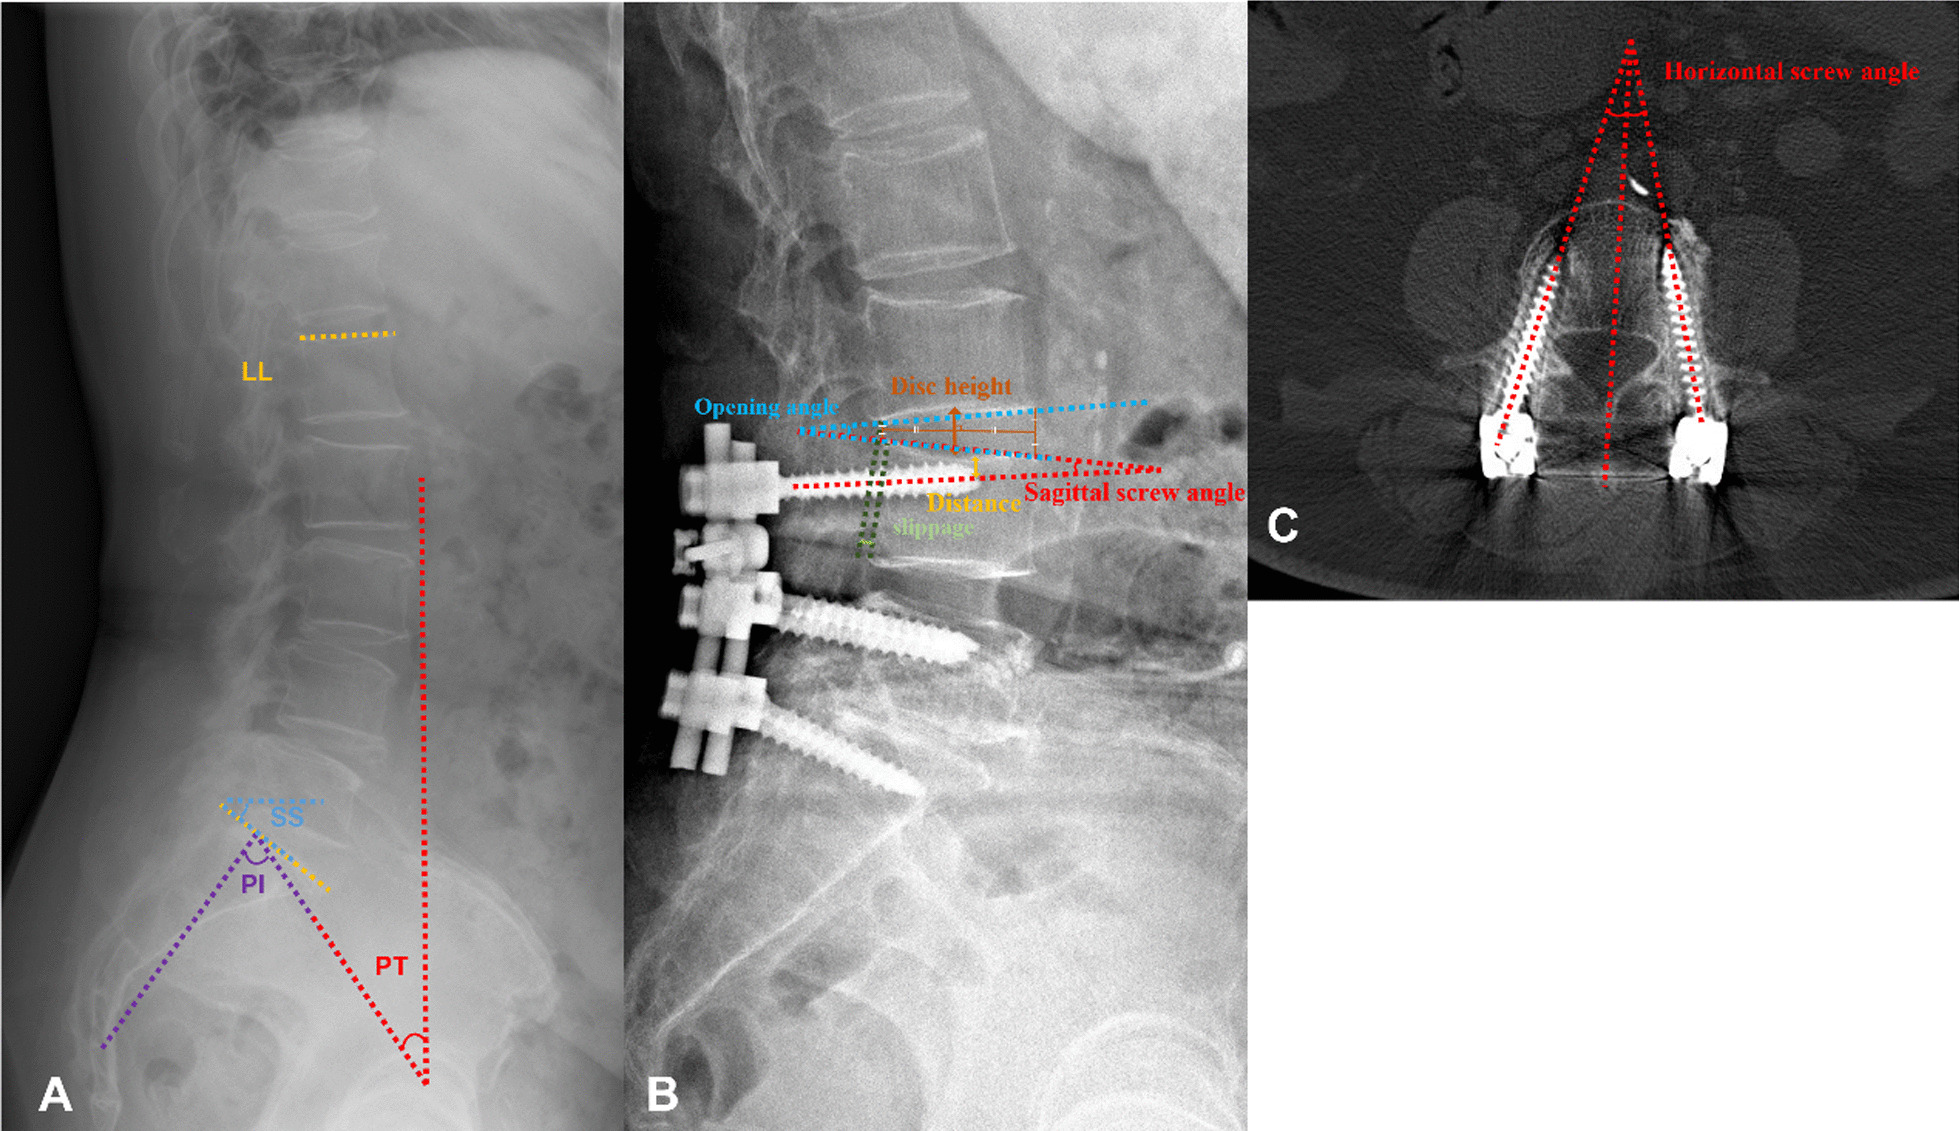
Parameters of radiological evaluation of spinopelvic parameters, ASD diagnosis and pedicle screw fixation. A Preoperative spinopelvic parameters of patients evaluated by lateral radiograph. B Proximal adjacent segment disk height, opening angle at L3–4, L3 vertebral slippage, distance of pedicle screw apex to superior endplate and sagittal screw angle between pedicle screw and superior endplate of L4 by lateral radiograph (not the same patient in A). C Horizontal screw angle, the mean angle of two pedicle screws at L4 and middle line by horizontal plane
